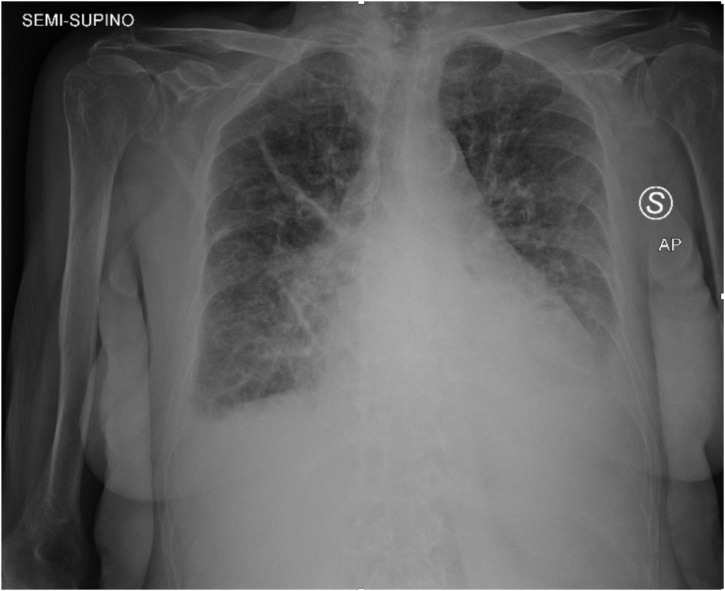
Chest X-ray was acquired in the semi-sitting position and showed bilateral diffuse pulmonary infiltrates consistent with pulmonary edema and bilateral pleural effusion.
